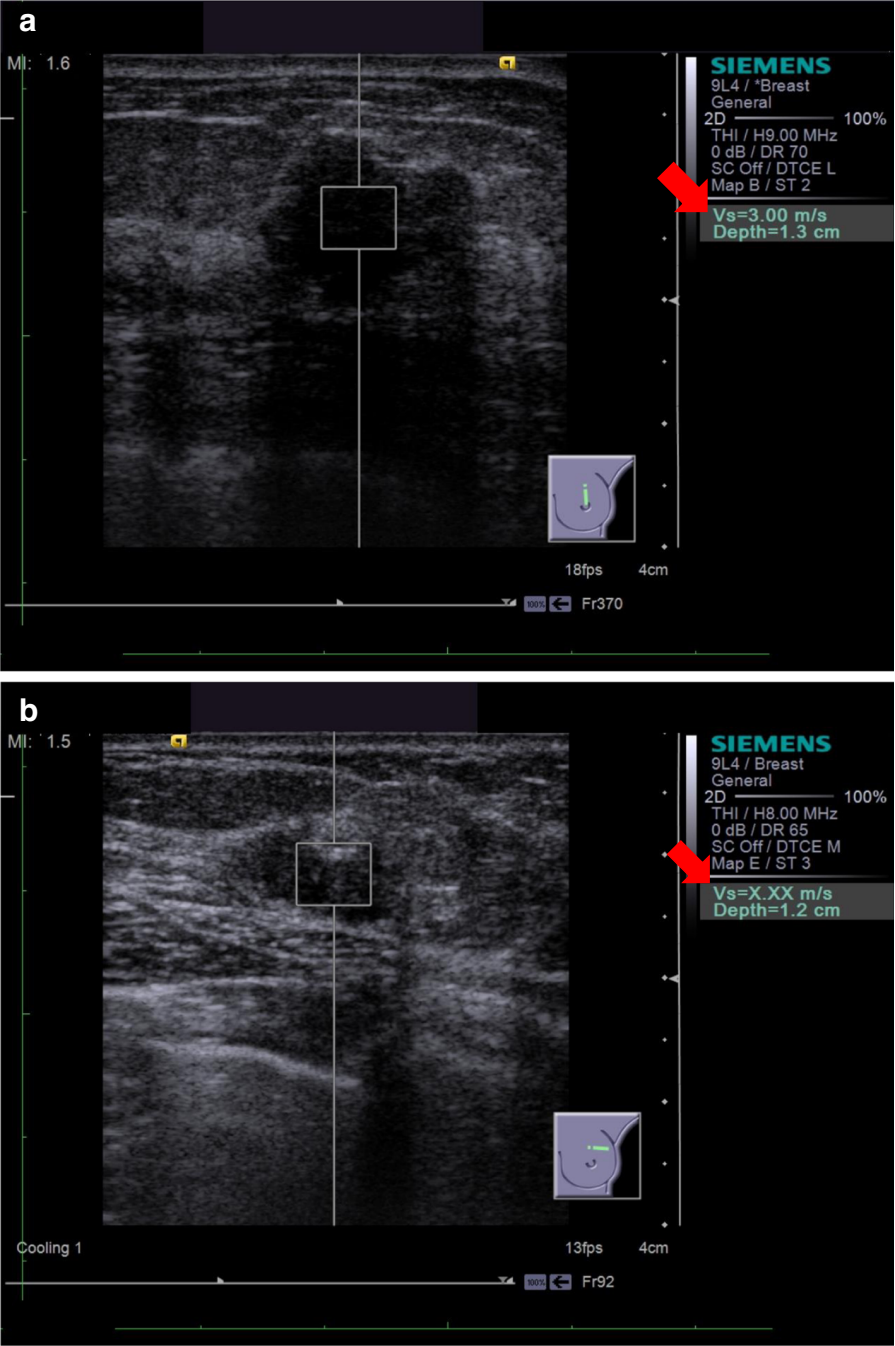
Typical Virtual Touch tissue quantification (VTTQ) images with results. During real-time ultrasonography, a 5 × 5-mm region of interest is placed on the area of interest. When VTTQ is activated, the display is frozen and the calculated VTTQ value is displayed (1a). When VVTQ is unsuccessful, ‘X.XXm/s’ is displayed (1b).
